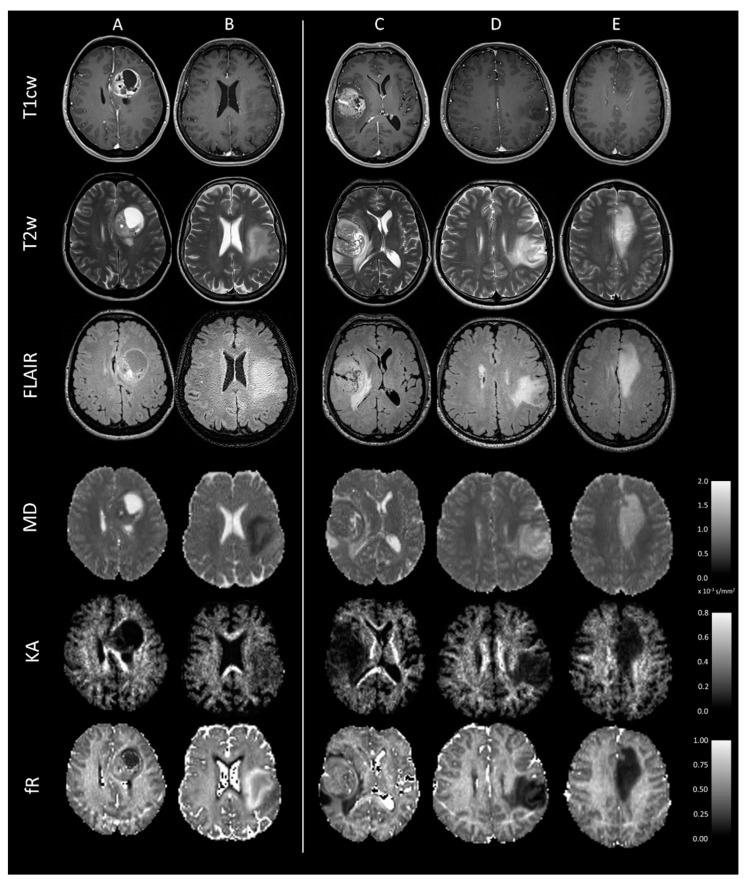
Axial T1cw, T2w, and FLAIR images and MD, KA, and fR maps at the level of the tumour are shown for five representative subjects with distinct molecular subtypes of adult-type gliomas. (A) Patient with IDH-wildtype glioblastoma: a necrotic central area is surrounded by a rim of enhancement following gadolinium injection with low MD and high KA and fR. This distinct radiologic appearance can help the network to distinguish this subtype from those with IDH-mutation. (B) Patient with molecular GBM: lack of enhancement and necrosis, but with low MD and high KA and fR. (C) Patient classified as grade 4 IDH-mutant astrocytoma according to WHO CNS5: radiological characteristics similar to an IDH-wildtype glioblastoma. (D) Patient with IDH-mutant astrocytoma: lack of enhancement and necrosis, with high MD and low KA and fR. (E) Oligodendroglioma with IDH-mutation and 1p/19q codeletion: lack or subtle contrast enhancement with high MD and low KA and fR. T1cw = post-contrast T1-weighted; T2w = T2-weighted; MD = mean diffusivity; KA = kurtosis anisotropy; fR = restricted fraction; GBM = glioblastoma; and WHO CNS5 = fifth edition of the WHO classification of the central nervous system tumours.
